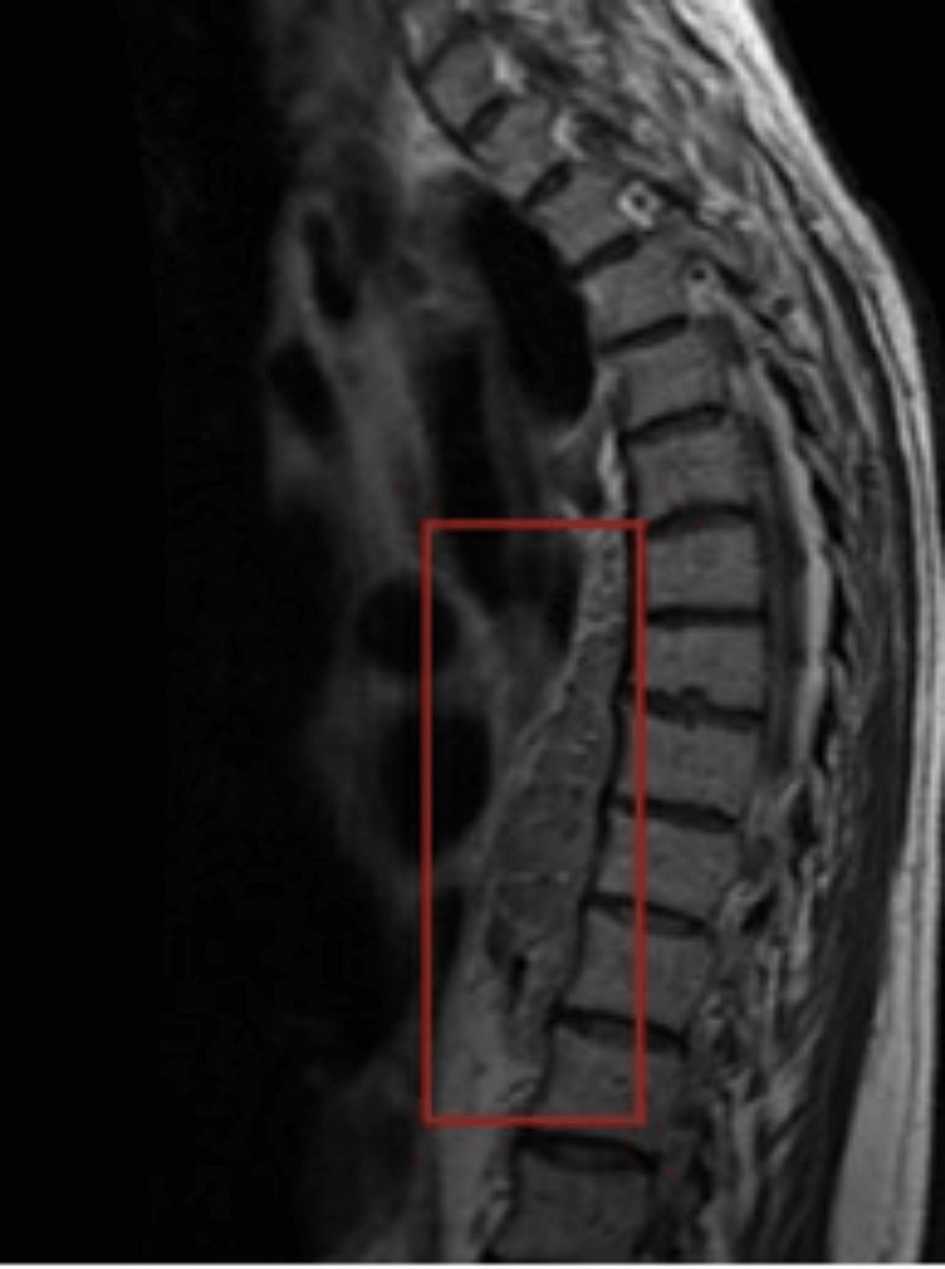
MRI lumbosacral region showing soft tissue mass
